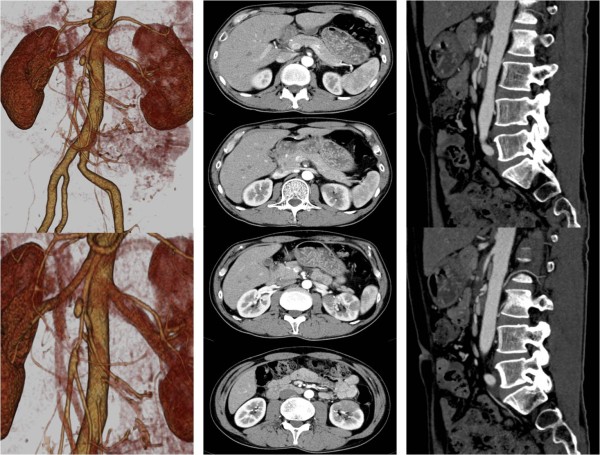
Computed tomography at the time of first examination (left, three-dimensional construction; middle, transverse plane; right, sagittal plane). A dissecting arterial aneurysm, with a maximum diameter of 12.3mm (compared with 6.6mm at an unaffected site), was localized approximately 1.5 and 5cm distal to the ostium of the superior mesenteric artery. A false lumen was partially occupied by a thrombus. Distal to the occlusion, the arterial wall was irregularly shaped, with narrow and wide sections. An irregular shaped arterial wall was also observed in the inferior mesenteric artery. Arterial dissection with thromboembolism was also observed in the left renal artery, accompanied by ipsilateral renal infarction.
